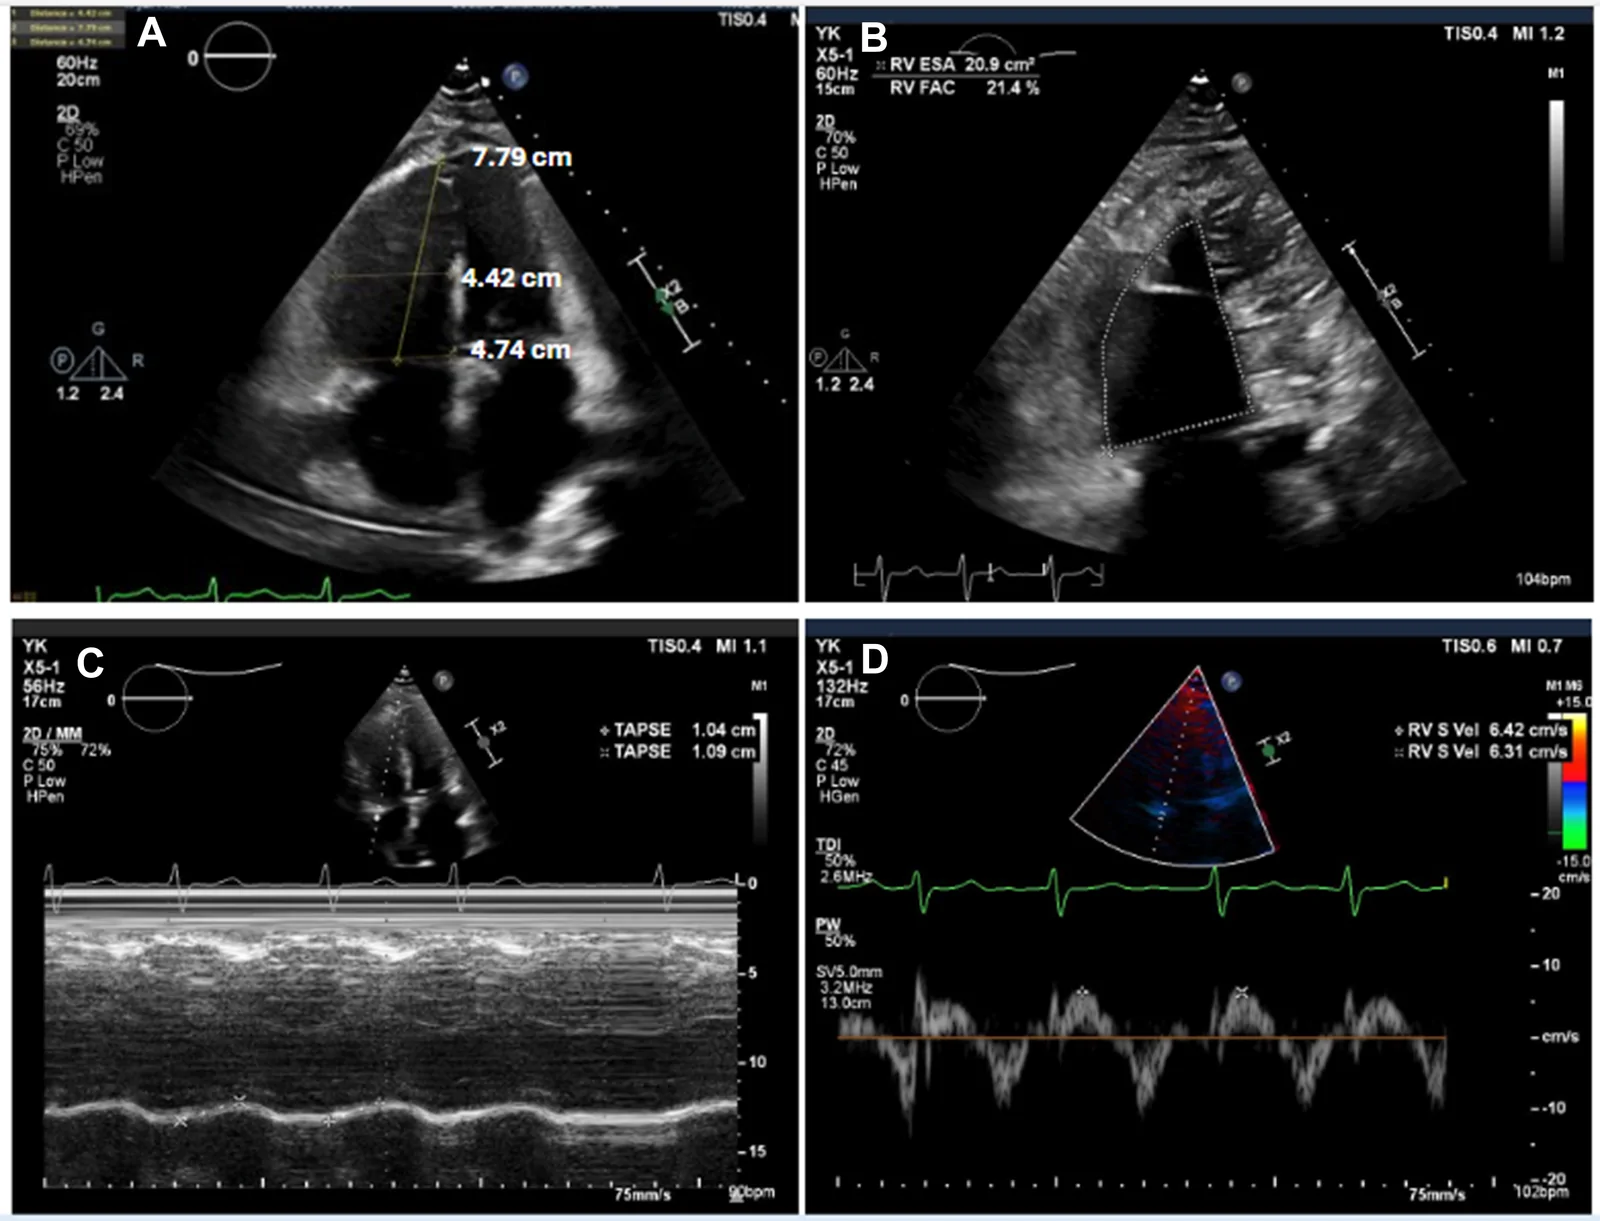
Right Ventricular Dysfunction at Baseline (A) RV-focused apical 4-chamber view on TTE demonstrating dilated right ventricle, with a mid-RV short-axis width of 4.42 cm and basal RV short-axis width of 4.74 cm. (B) RV-focused apical 4-chamber view on TTE demonstrating fractional area change of 21.4%, consistent with dilated RV. (C) TAPSE in M-mode is reduced at 1.04 cm, consistent with reduced RV systolic function. (D) Pulse-wave Doppler of the RV septum on TTE demonstrating reduced RV S′ of 6.31 cm/s, consistent with reduced RV systolic function. RV = right ventricular; TAPSE = tricuspid annular plane systolic excursion; TTE = transthoracic echocardiography.
